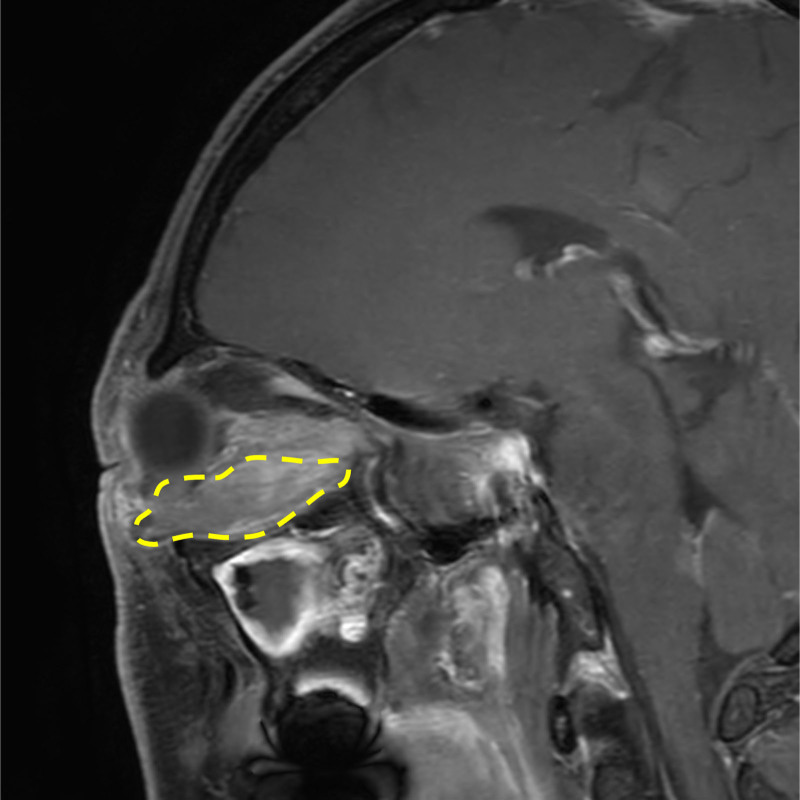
Sagittal T1-weighted image with contrast shows a 3 × 2 cm retrobulbar mass with homogeneous enhancement.
